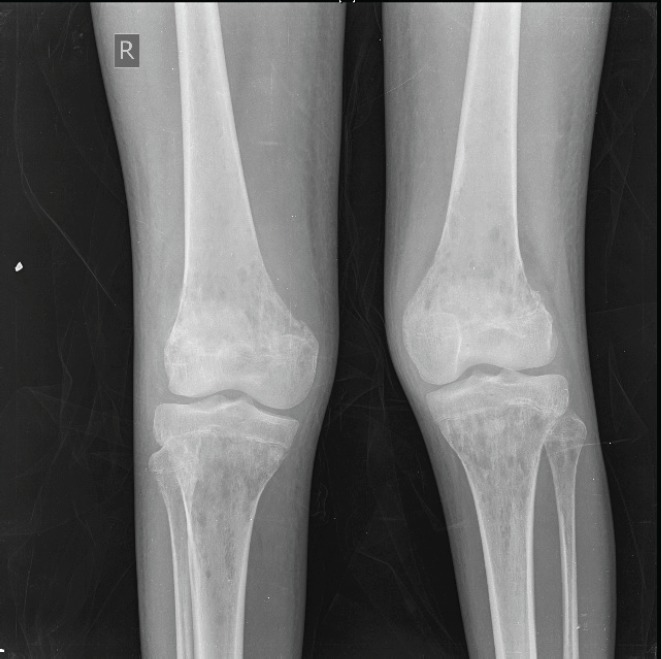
X-ray of the knee joint showing multiple lytic in the bones around the knee joint bilaterally.
